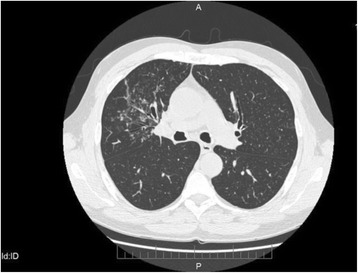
HRCT. Ground glass consolidation in anterior segment of right upper lobe
